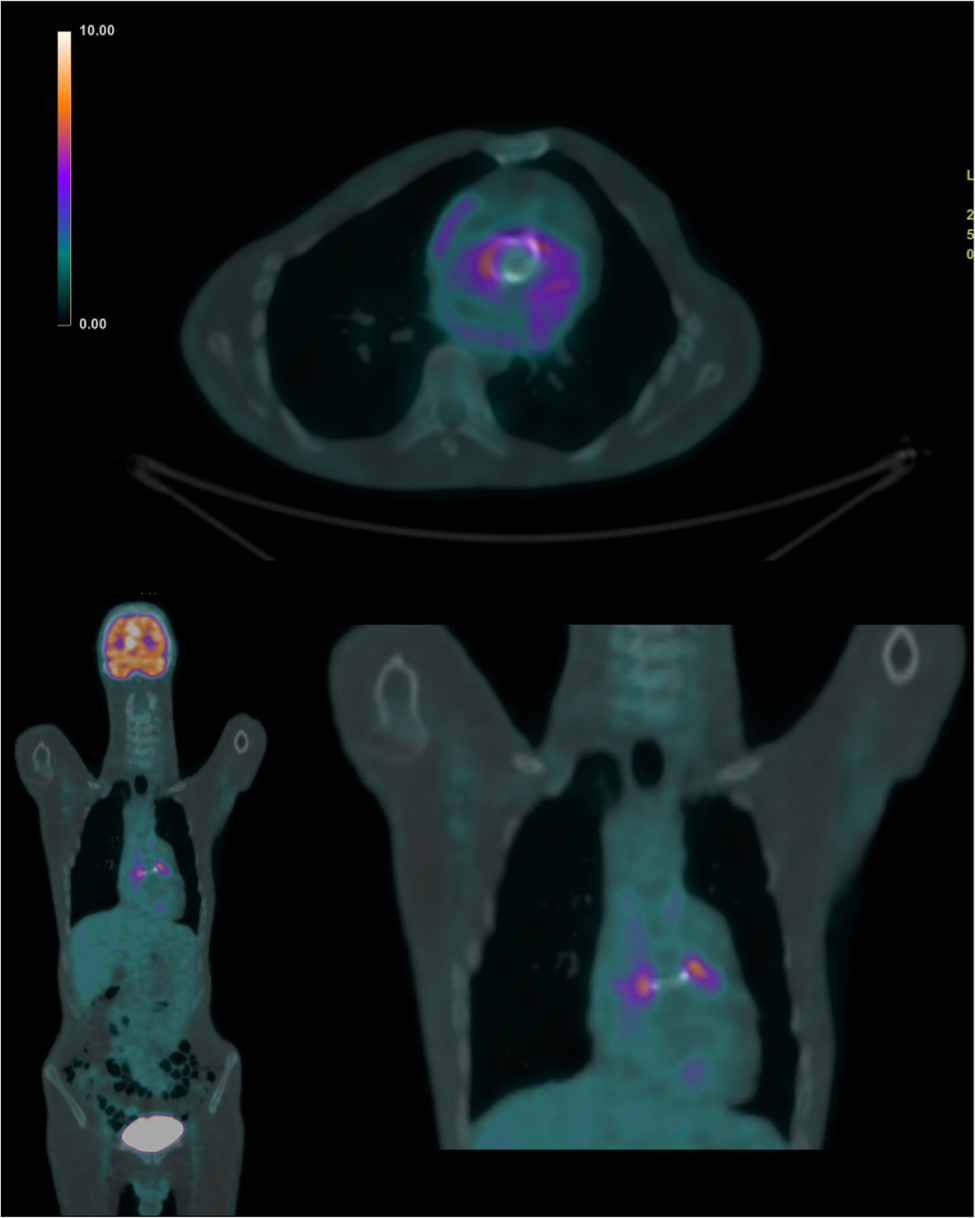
Positron emission tomography–computed tomography showing focal and diffuse uptake of the radiometabolic tracer around the tissue in the periaortic site
